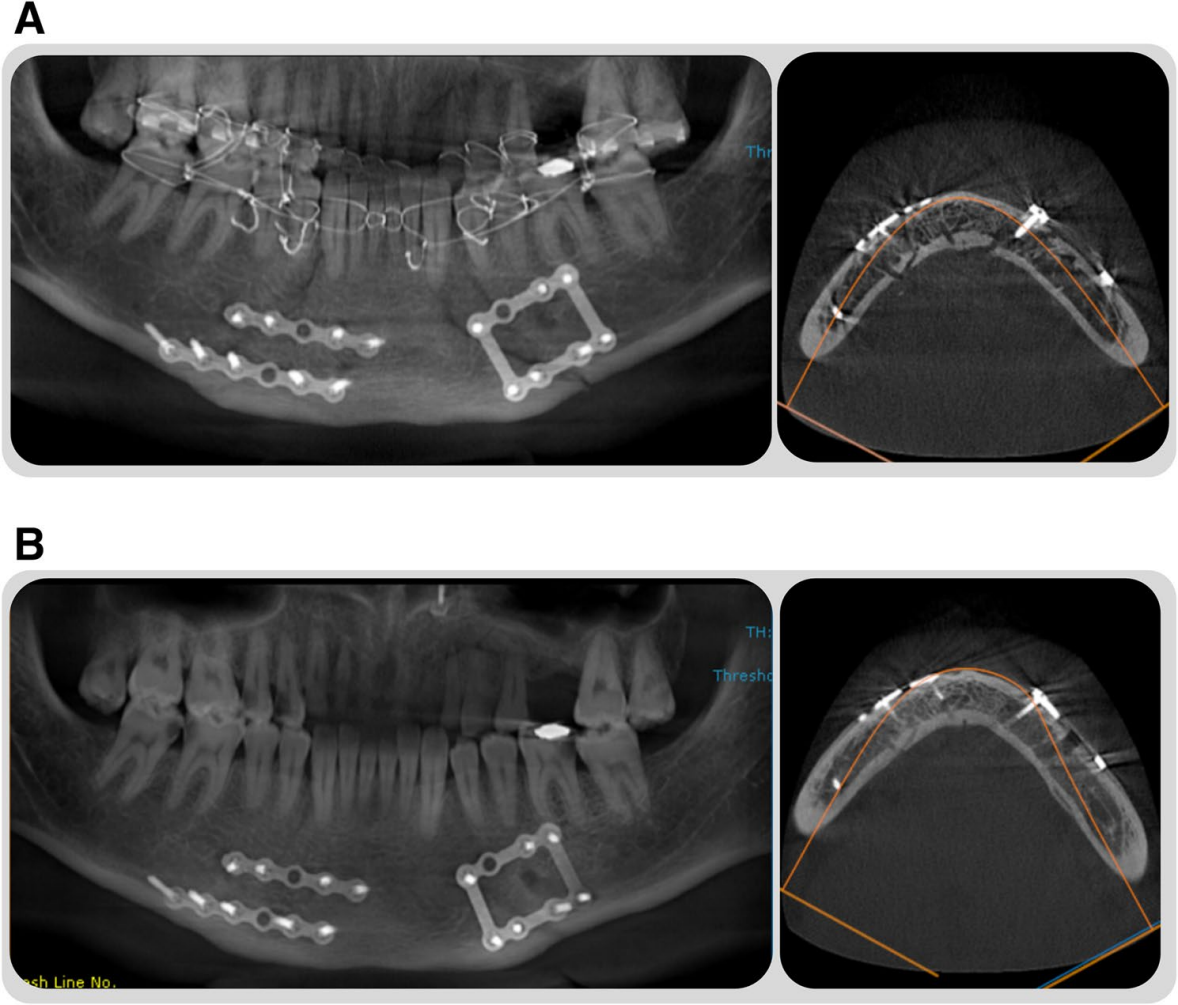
Postoperative radiographic assessment. A, Immediate postoperative CBCT scan. B, 12-week postoperative CBCT scan
